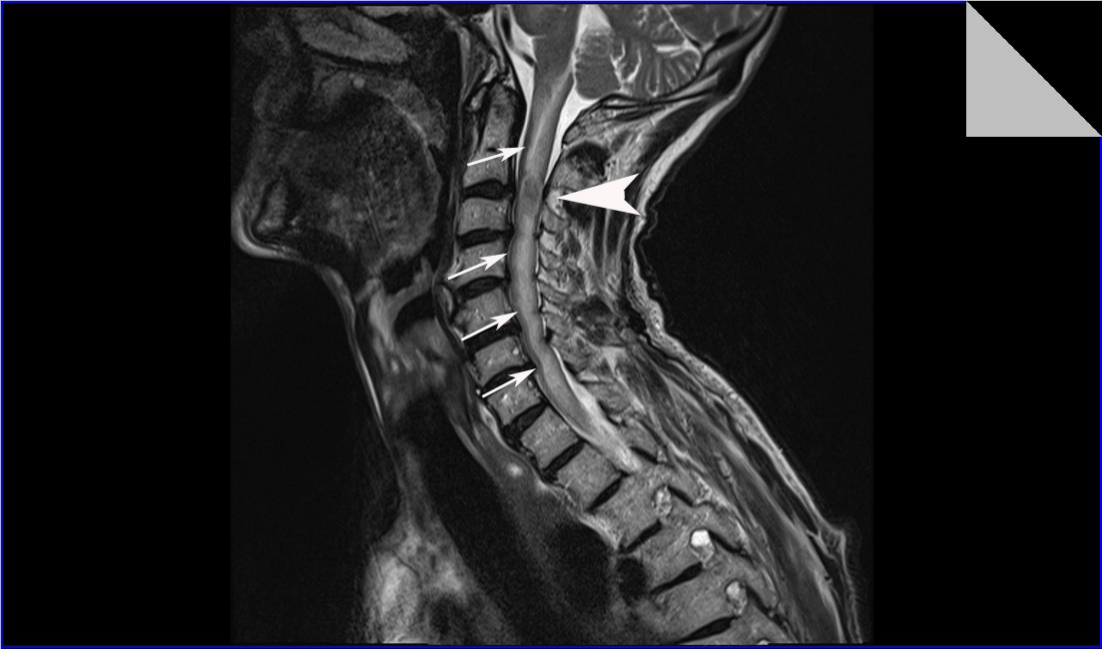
Sagittal T2-weighted MRI of his cervical spine shows an oblong expansile intramedullary lesion at the C2-C3 level of his spinal cord, associated with large proximal and distal syrinx and edema extending from the cervico-medullary junction to the inferior aspect of the T3 vertebral body.
